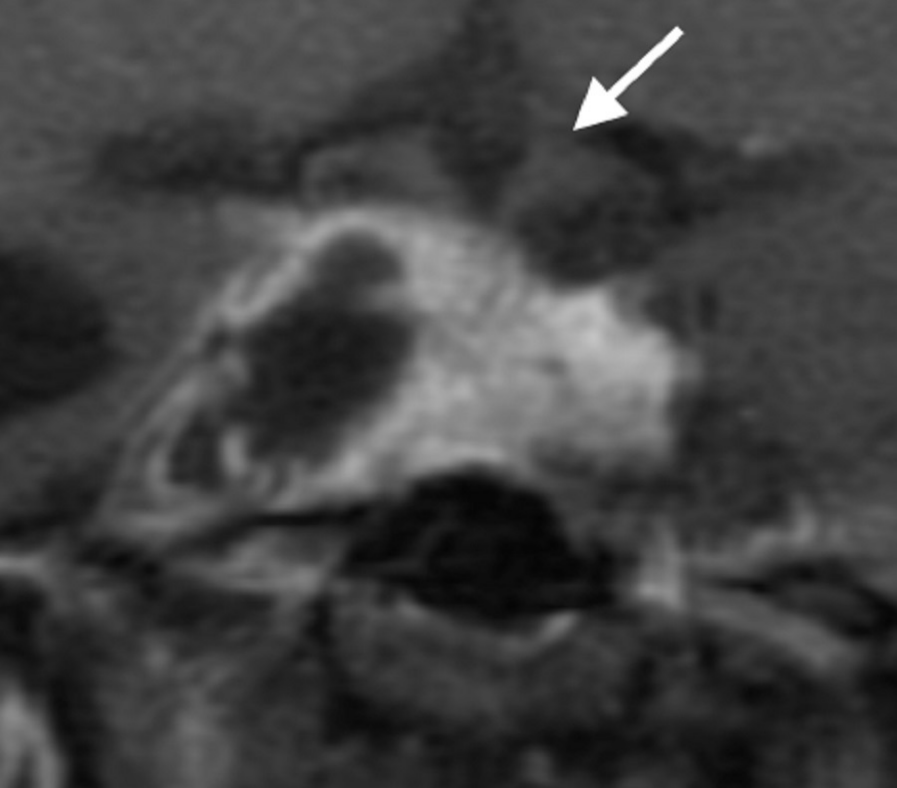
Coronal Post-Contrast MRI Sequences After Cyberknife radiosurgery
